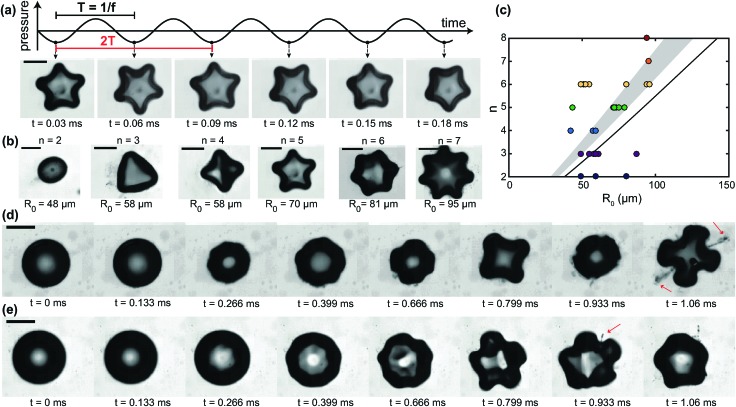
Shape oscillations of particle-coated bubbles and directional particle expulsion. (a) A particle-coated bubble undergoing shape oscillations with mode *n* = 5. The period of the ultrasonic driving is *T* = 1/*f*; the period of the shape oscillation is 2*T*, corresponding to a frequency *f*/2. (b) Examples of shape oscillations of particle-coated bubbles with different modes *n* observed in experiment. *R*
